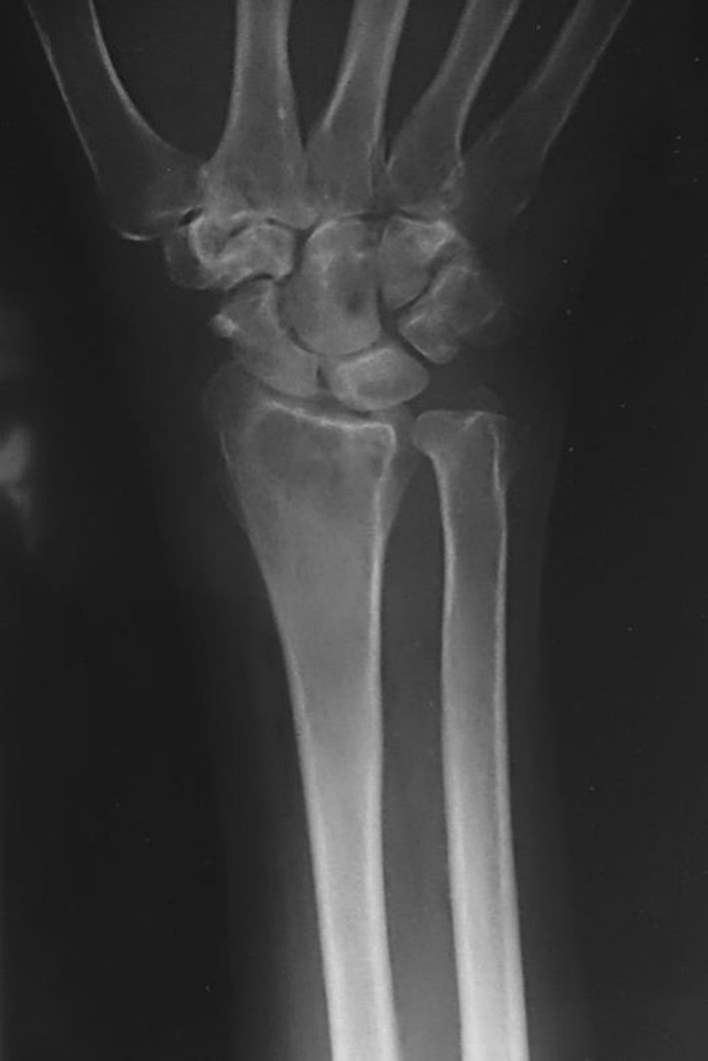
X-ray of the distal radius/ulna with the wrist showing localized osteopenia around the radiocarpal joint
